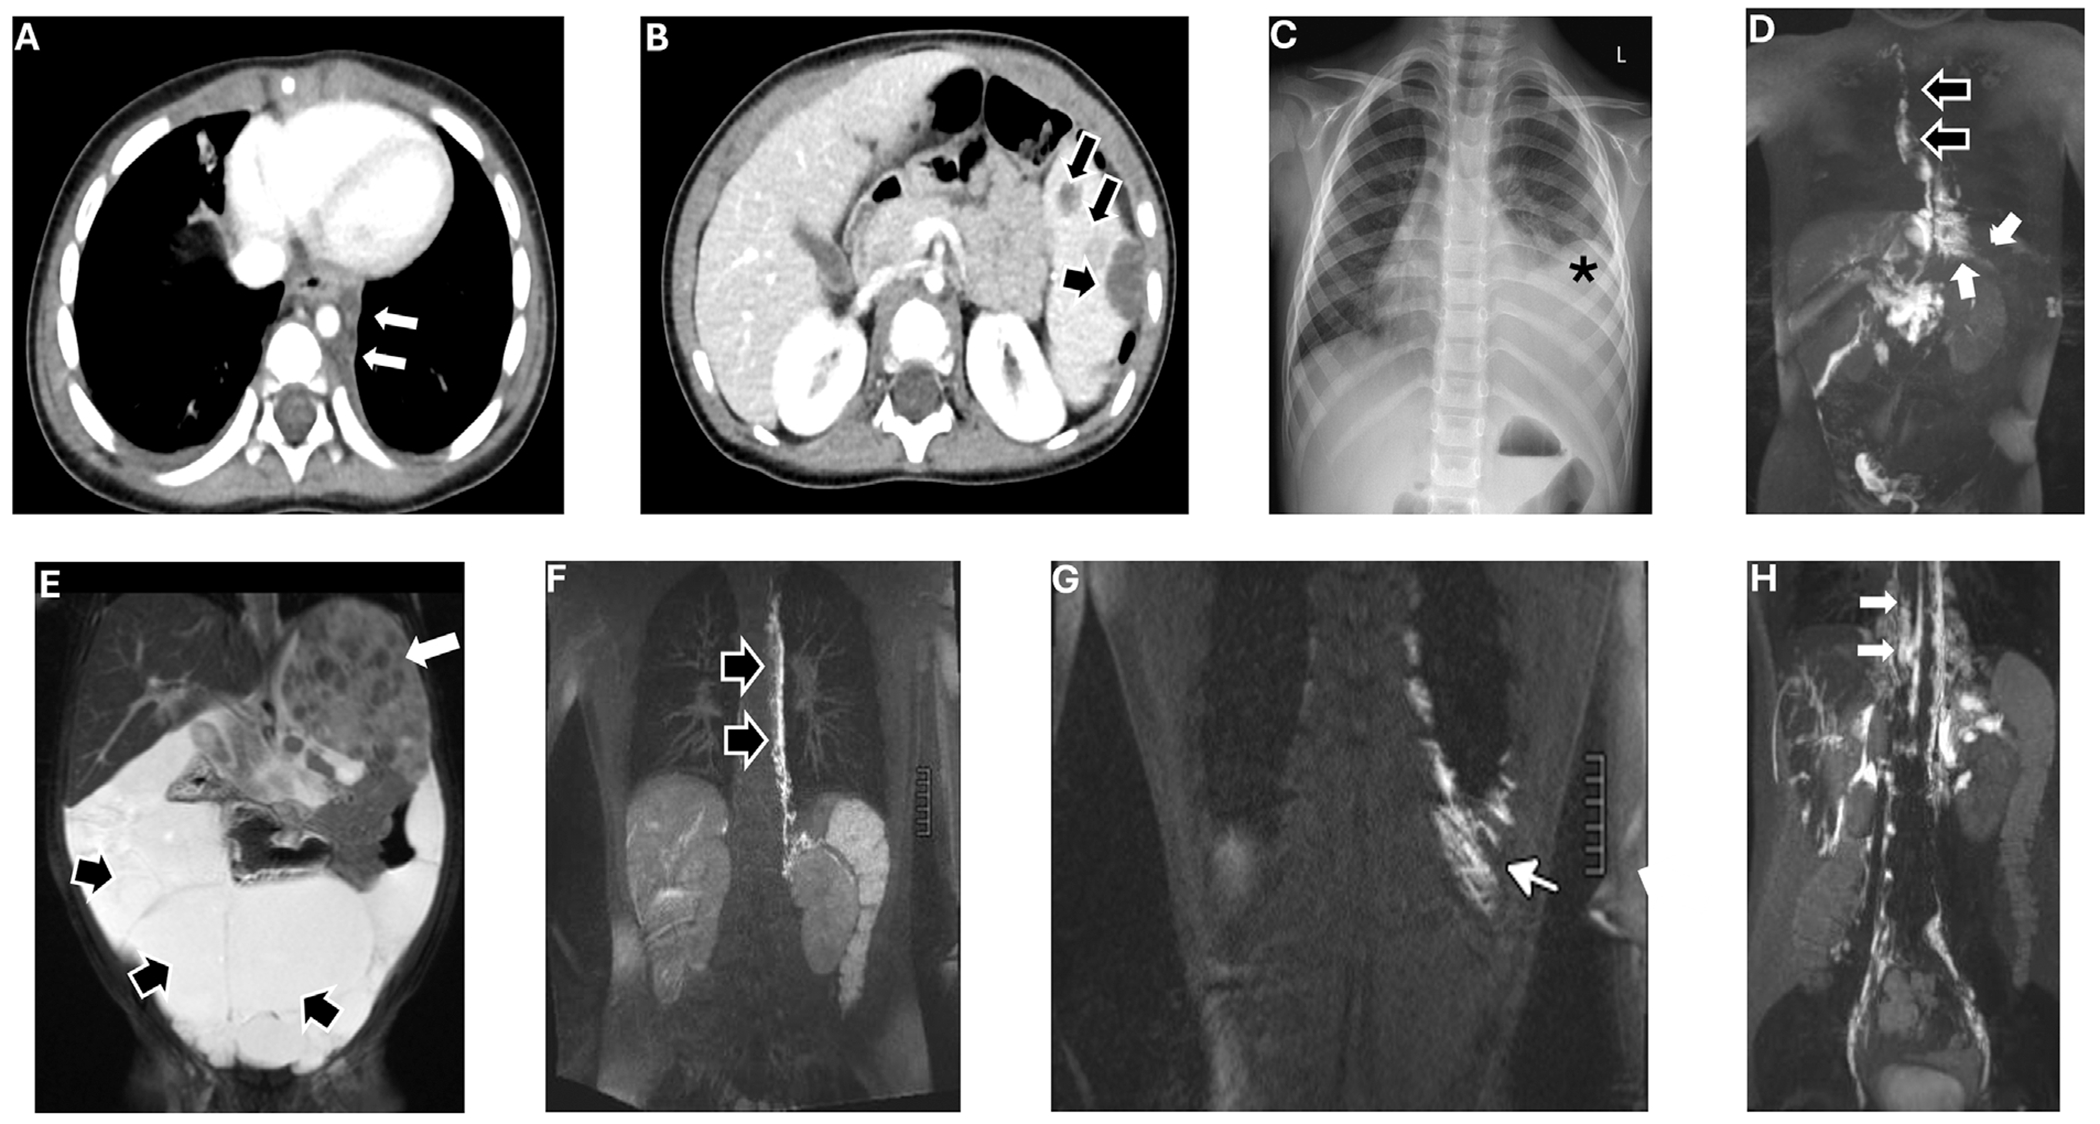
Imaging for Cases 3 and 4. (A–D) Patient 3: 4-year-old male with generalized lymphatic anomaly (GLA). (A and B) Computed tomography (CT) shows paraspinal lymphatic malformation (white arrows) and lymphatic cysts in the spleen (black arrows). (C) Chest radiograph demonstrates a large left pleural effusion (asterisk). (D) Magnetic resonance lymphangiography (MRL) after hepatic injection shows opacification of the paraspinal lymphatic malformation, with associated intercostal perfusion (white arrows), along with visualization of the right-sided thoracic duct (black arrows). (E–H) Patient 4: 21-year-old with GLA with abnormal lymphatic conduction. (E) Magnetic resonance imaging (MRI) of a toddler demonstrates multilocular lymphatic malformation of the abdomen (black arrows) with splenic involvement (white arrow). (F and G) MRL after liver injection shows collateral lymphatic channels along the left paravertebral network (black arrows) and intercostal perfusion (white arrow). (H) MRL with groin injection demonstrates dilated collateral lymphatic channels of the mediastinum (white arrows).
